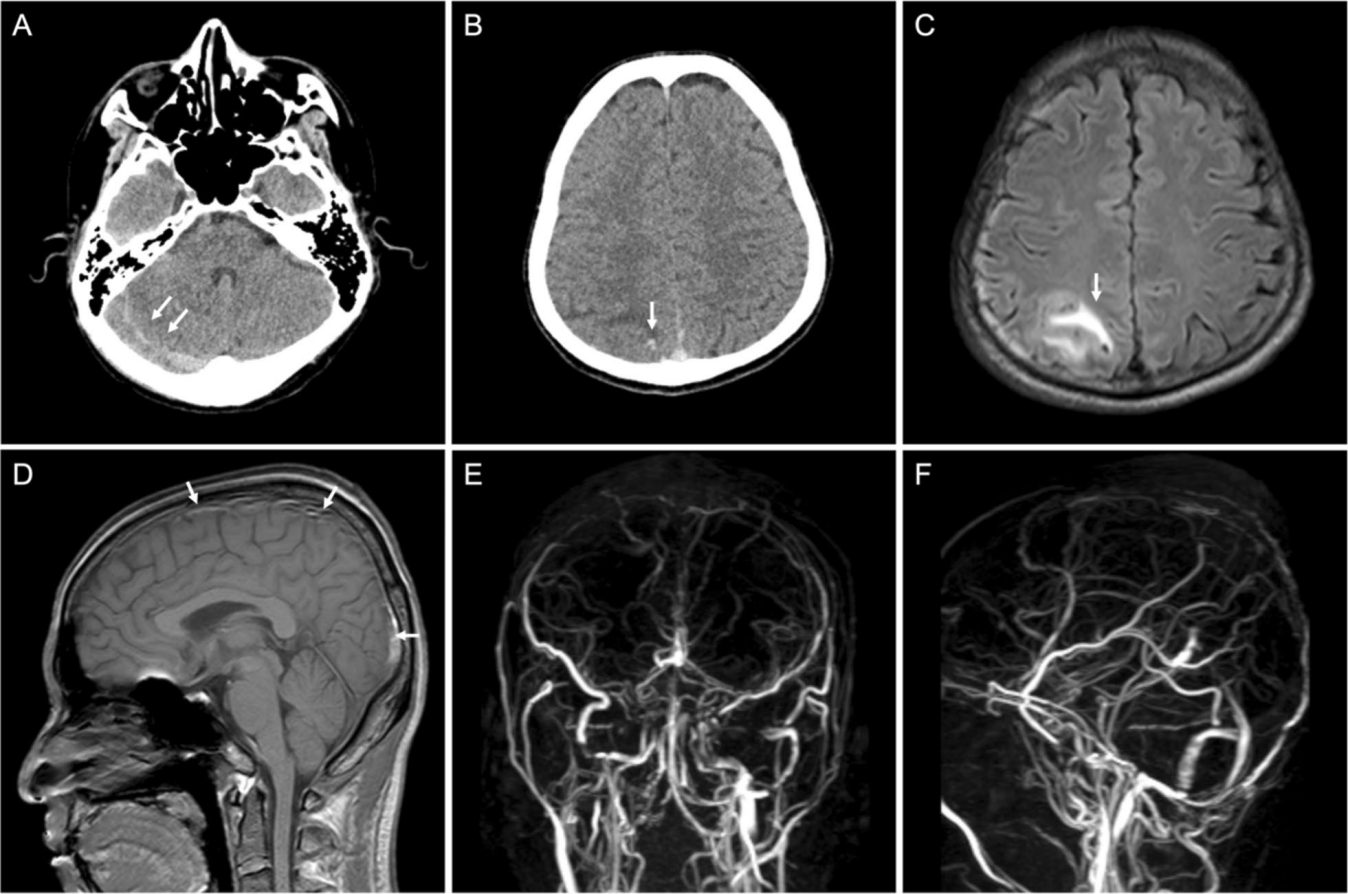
Non-contrast head CT demonstrating SDH of the posterior fossa with an approximate diameter of 0.6 cm (A), and small parasagittal ICH of the right parietal lobe surrounded by perifocal edema (B). Head MRI: FLAIR sequence reveals perifocal edema surrounding the site of hemorrhage (C), and T1-weighted MRI reveals thrombosis of the superior sagittal sinus (D). Head MRV (phase-contrast technique) demonstrates thrombosis of the superior sagittal sinus, right transverse sinus, right sigmoid sinus, and right sinus of trolard (E, F)
